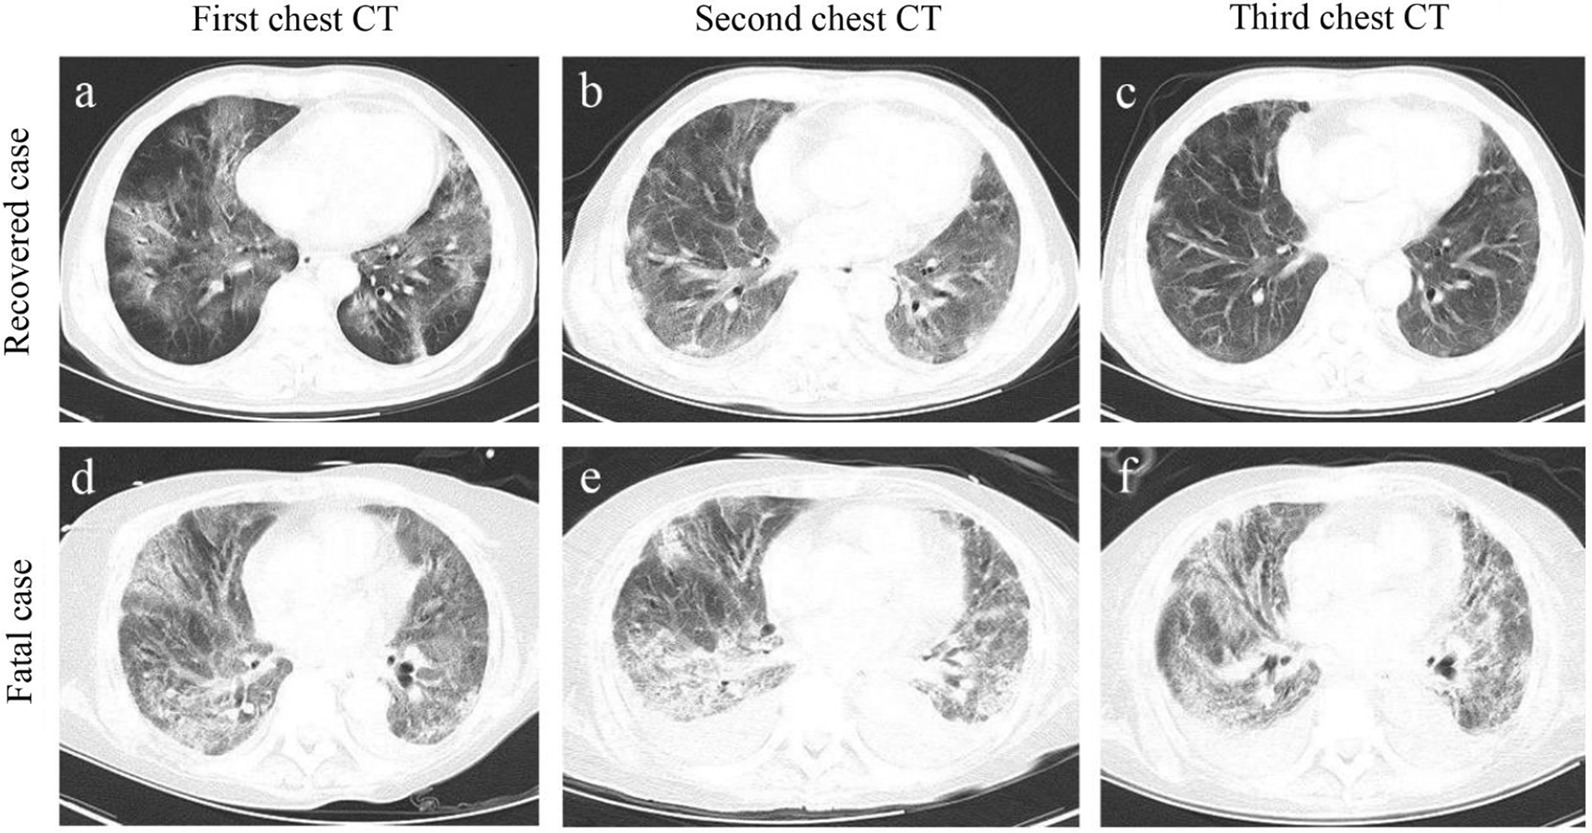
Representative chest computed tomographic images of fatal and recovered patients with COVID-19. a-c. An elderly male recovered case confirmed with COVID-19 at different disease stages. a Axial chest CT image obtained at the onset shows diffuse ground-glass opacity (GGO) and fibrous stripes; b axial chest CT image obtained at the middle stage shows bilateral, peripheral GGO and fibrous stripes associated with smooth interlobular and intralobular septal thickening; c axial chest CT image obtained at discharge stage shows bilateral fibrous stripes and nodules. d–f An elderly male fatal case confirmed with COVID-19 at different disease stages. a Axial chest CT obtained at the onset shows bilateral diffuse GGO associated with round cystic change, local bronchial meteorology and left pleural effusion; e axial chest CT image obtained at the middle stage shows the progression of bilateral GGO, bronchial meteorology and pleural effusion; f axial chest CT image obtained near stage of death shows the progression of bilateral GGO, round cystic change, bronchial meteorology, and increase of pleural effusion
